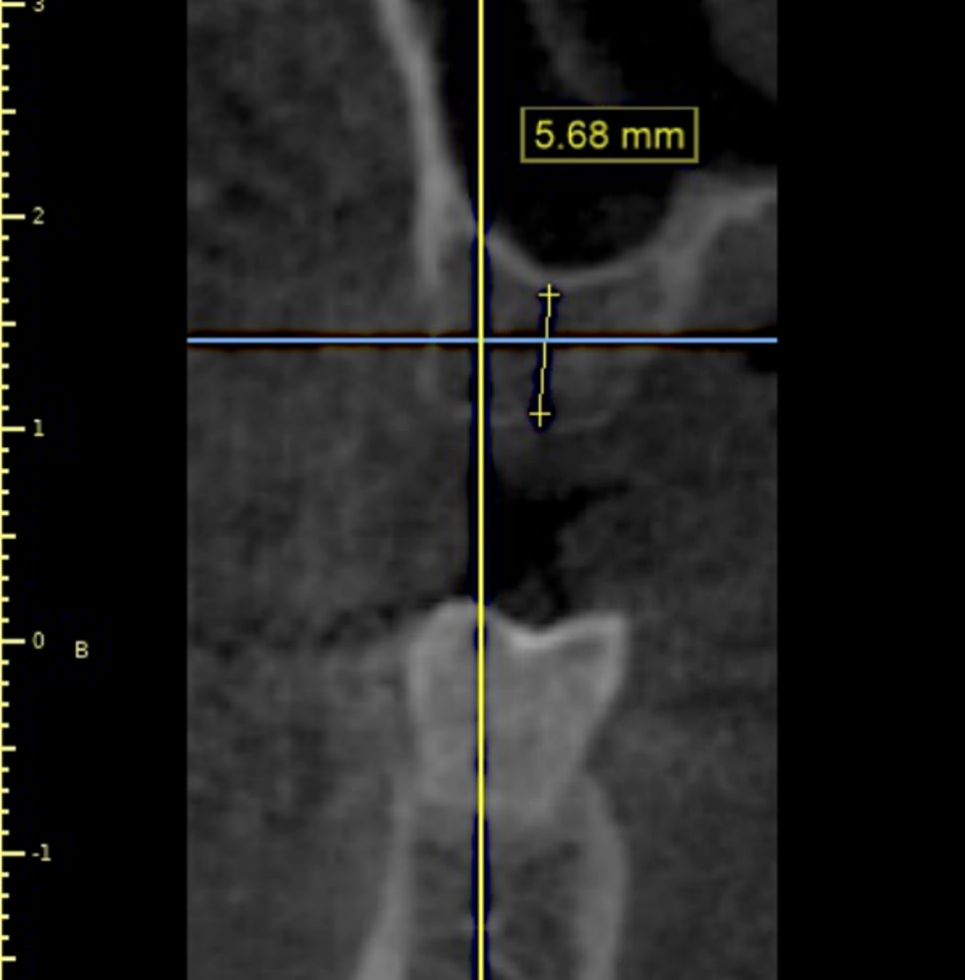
Sections of reformatted sagittal cut from the preoperative CBCT to assess presence of any sinus pathosis and to accurately measure the residual alveolar bone height
